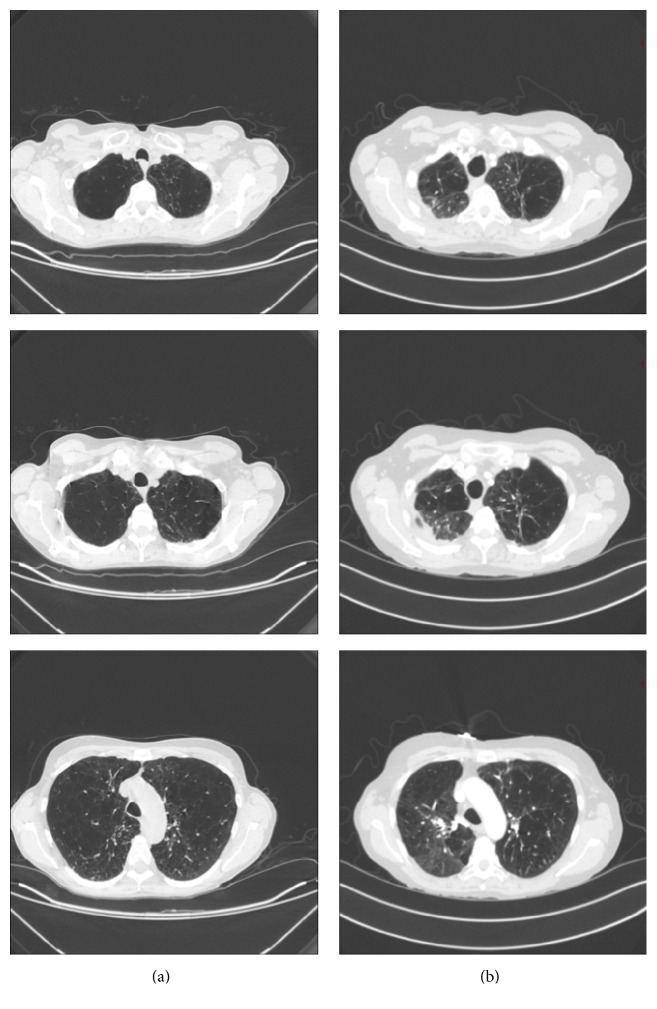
Baseline CT scan (a) which shows diffuse emphysema and a follow-up CT (b) which shows apical scarring predominantly on the right and right apical pleural thickening.
